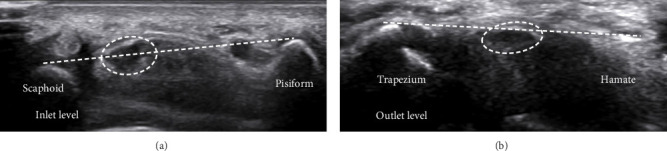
Ultrasound images of the median nerve at key anatomical landmarks. (a) The inlet level, showing the median nerve positioned between the scaphoid and pisiform bones. (b) The outlet level, demonstrating the median nerve near the trapezium and hamate bones.
